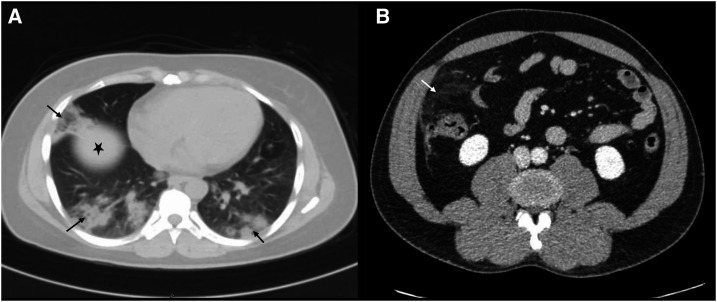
(A) Abdominal computed tomography (CT) scan of case 1 showing lower lung zones with patchy bilateral areas of consolidation and ground-glass attenuation (black arrows). Liver (star). (B) CT scan of the abdomen of case 2 showing diffuse stranding of the right hypochondrial omentum/mesentery surrounding a focal fat dense ovoid area (white arrow) suggestive of an underlying epiploic appendagitis.
